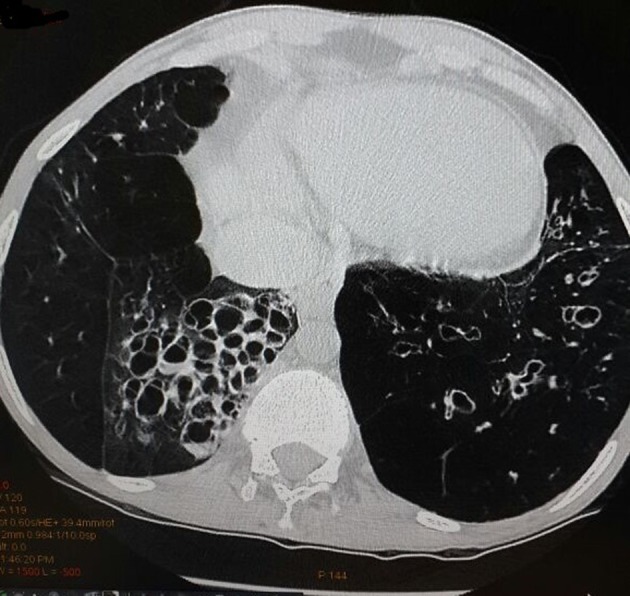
High-resolution computed tomography scan with cystic bronchiectasis showing bronchial dilation, lack of bronchial tapering and visibility of airways within 1 cm of the pleural surface (abutting the mediastinal pleural surface)
